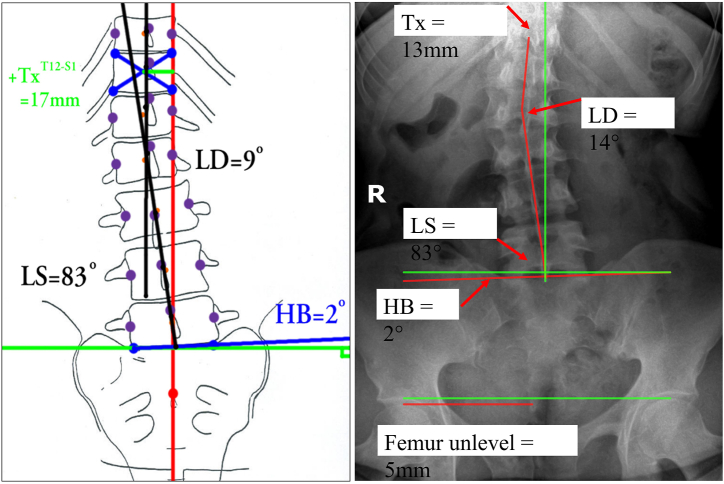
Spine radiographs were analyzed for: 1) a lateral translation of T12 compared to S2 tubercle (TxT12-S1), 2) a mid-lumbar angle (LD), 3) the sacral base to horizontal (HB), and 4) lateral bending of the lower lumbar vertebra compared with the sacral base (LS). This method has excellent reliability [44].
